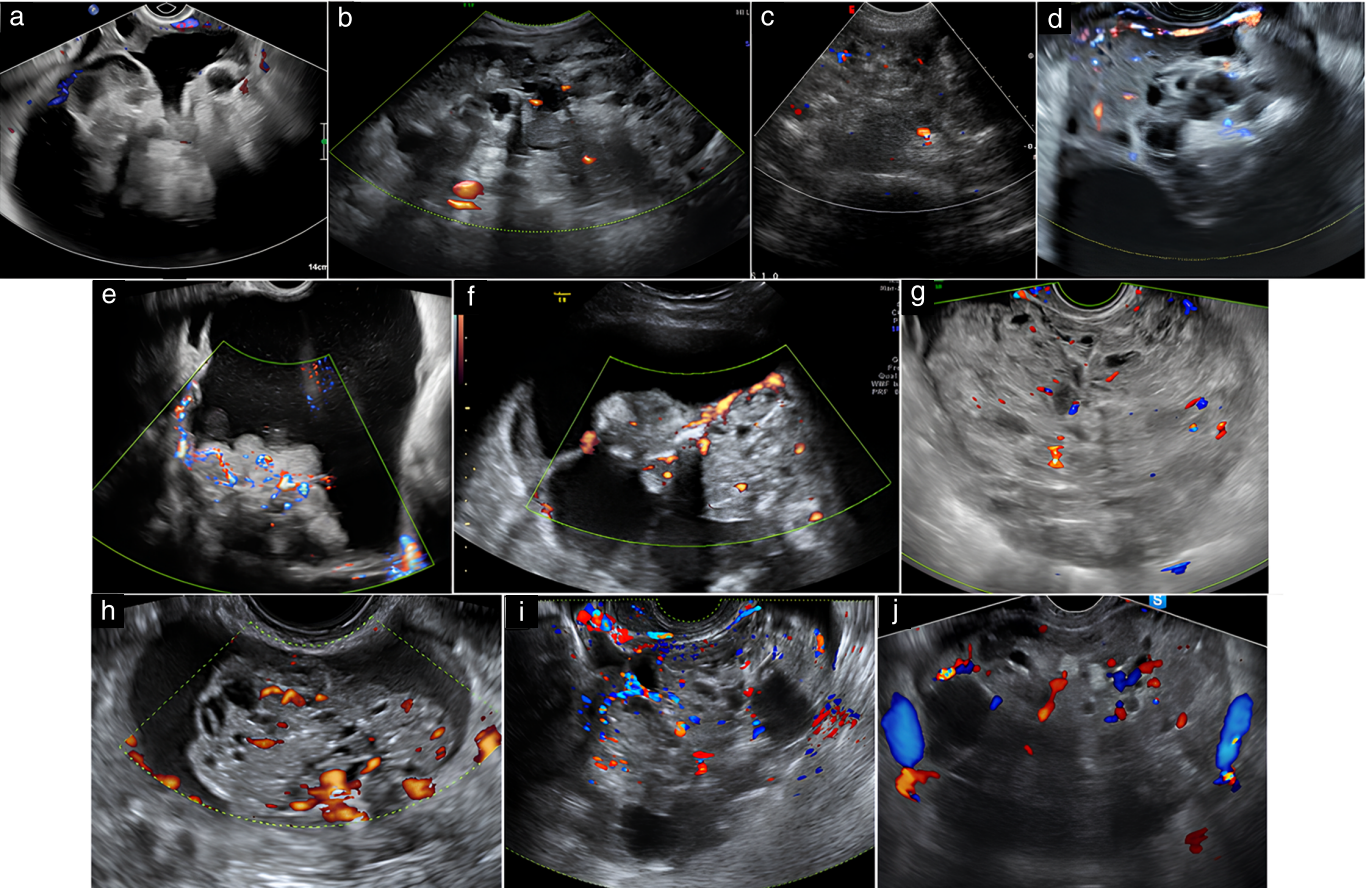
Color (a,c,d,e,g,i,j) and power (b,f,h) Doppler ultrasound images of 10 ovarian immature teratomas showing minimal (a–c) or moderate (d–j) vascularization. Pulse repetition frequency was 0.3 or 0.6 kHz.
